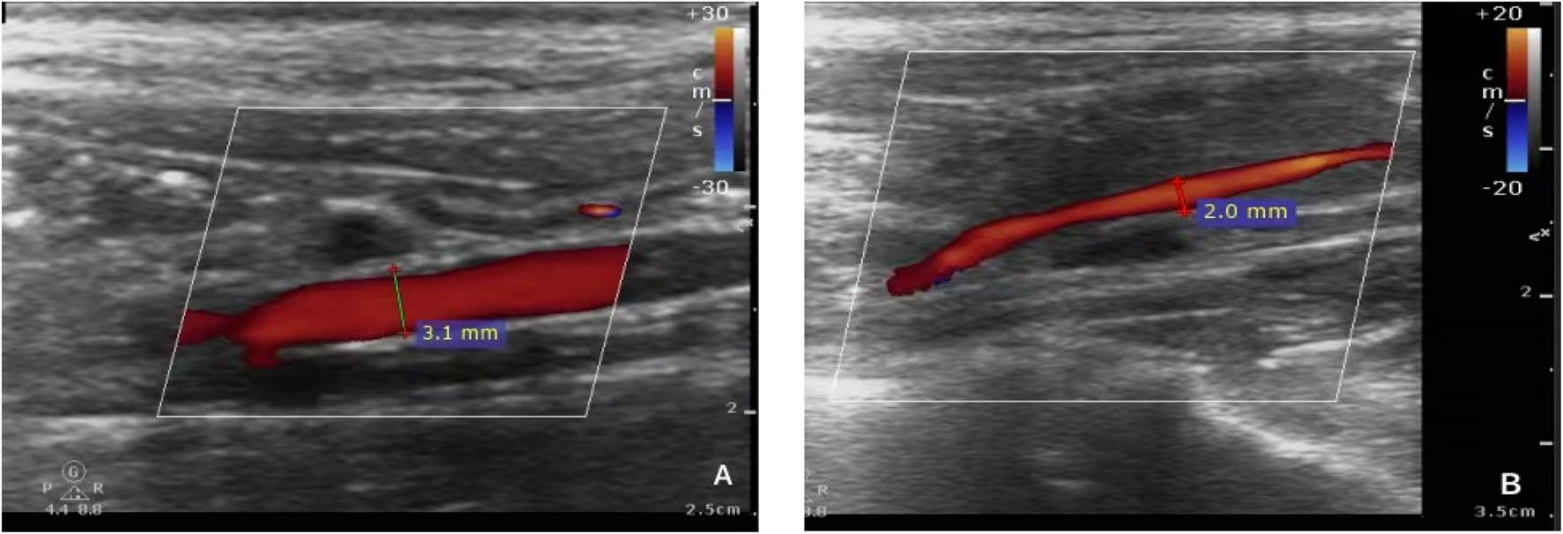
Changes in iliac artery blood flow before and after modeling. A: Before modeling, the internal diameter of the iliac artery was 0.31 cm. Color Doppler ultrasound showed the blood flow of the iliac artery. B: After free bleeding for the 30 s, color Doppler ultrasound showed that the artery rapidly collapsed with less blood flow and the inner diameter of the artery narrowed. The internal diameter of the iliac artery was 0.20 cm. And color Doppler ultrasound showed that the iliac vein was compressed and there was no blood flow signal
